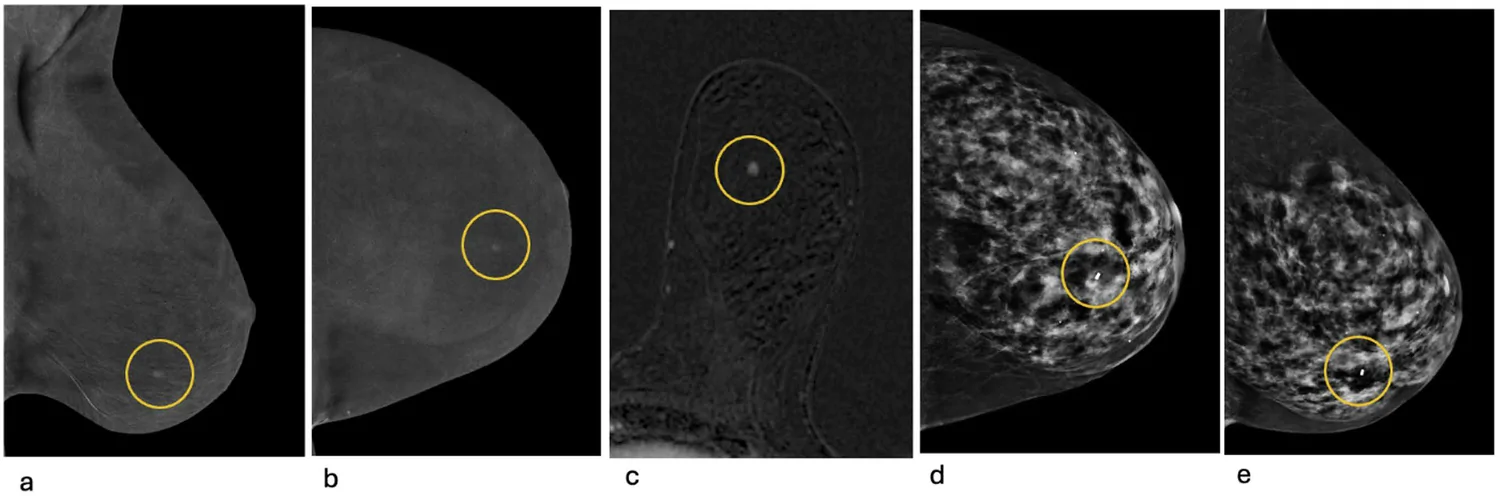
Low-conspicuity enhancing invasive carcinoma (not otherwise specified). A 76-year-old woman with a family history of breast cancer underwent routine screening CEM. On CEM, a low-conspicuity enhancing mass is seen on the recombined images, on both mediolateral oblique (a) and craniocaudal (b) projections, without a correlate on the low-energy images (not pictured). Subsequent contrast-enhanced MRI (c axial post-contrast subtraction sequence) demonstrated a corresponding enhancing mass. MRI-guided biopsy was performed, yielding invasive ductal carcinoma. Post-procedure full-field digital mammogram (d craniocaudal projection and e lateral projection) demonstrated the biopsy marker in the location of the originally identified enhancing mass
